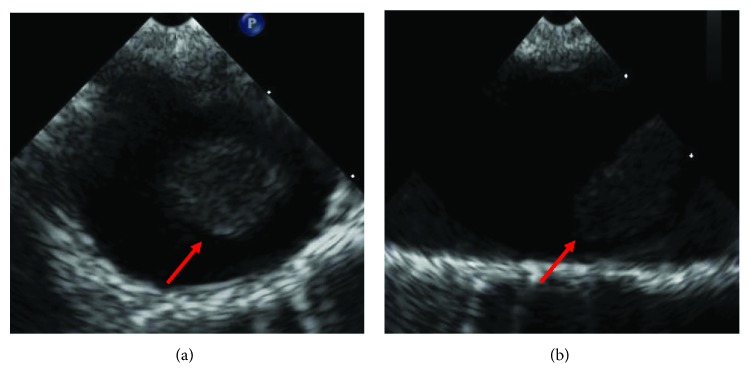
(a) Transesophageal echocardiogram (transverse view) of a large mass attached to the wall of the descending thoracic aorta. (b) Transesophageal echocardiogram (longitudinal view) of the large mobile mass attached to the aortic wall.
